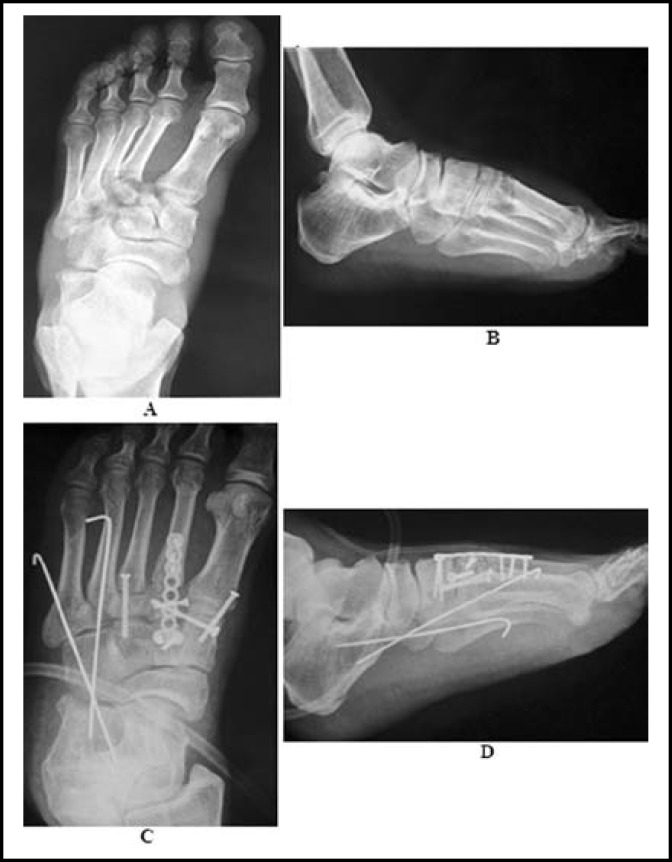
A male patient with Left foot total tarsometatarsal joint fracture dislocation caused by a traffic accident. (A, B) The X-ray showed a Myerson type A injury. (C, D) The postoperative X-ray showed a good reduction and fixation
